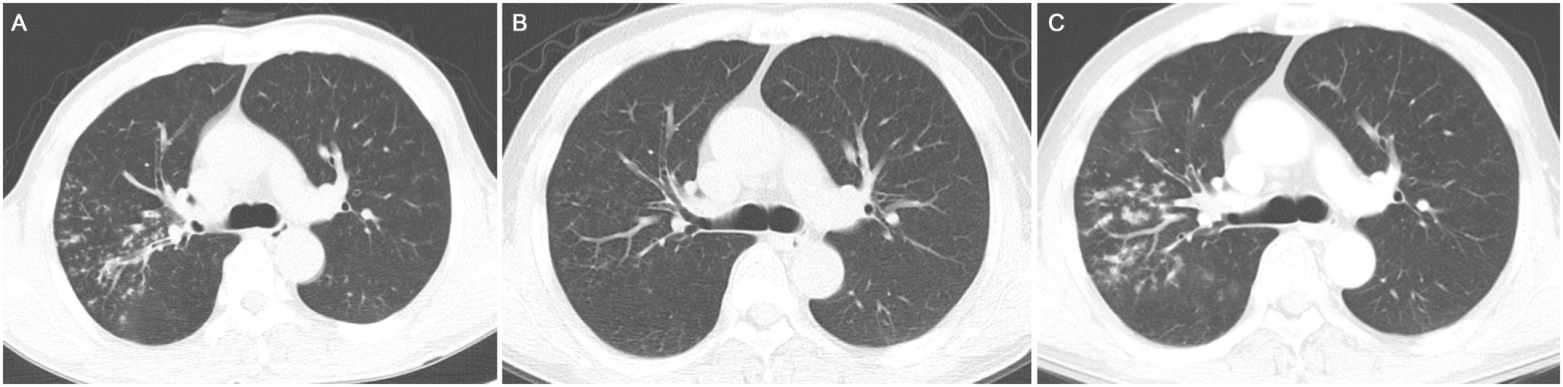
The changes in radiological imaging of one patient who experienced spontaneous conversion and reversion. (A) Chest computed tomography at the time of diagnosis of a 55 year-old man with *Mycobacterium abscessus* complex lung disease showing irregular centrilobular nodules with segmental distribution in the right upper lobe. (B) Follow-up chest computed tomography conducted approximately 16 months after diagnosis showing markedly improved radiological changes. The patient did not receive any treatment during the follow-up period and his sputum examination revealed spontaneous conversion. (C) Approximately 2 years later after spontaneous conversion, chest computed tomography showing redevelopment of centrilobular nodules in the right upper lobe. The growth of *Mycobacterium abscessus* complex was noted in the follow-up sputum examination.
